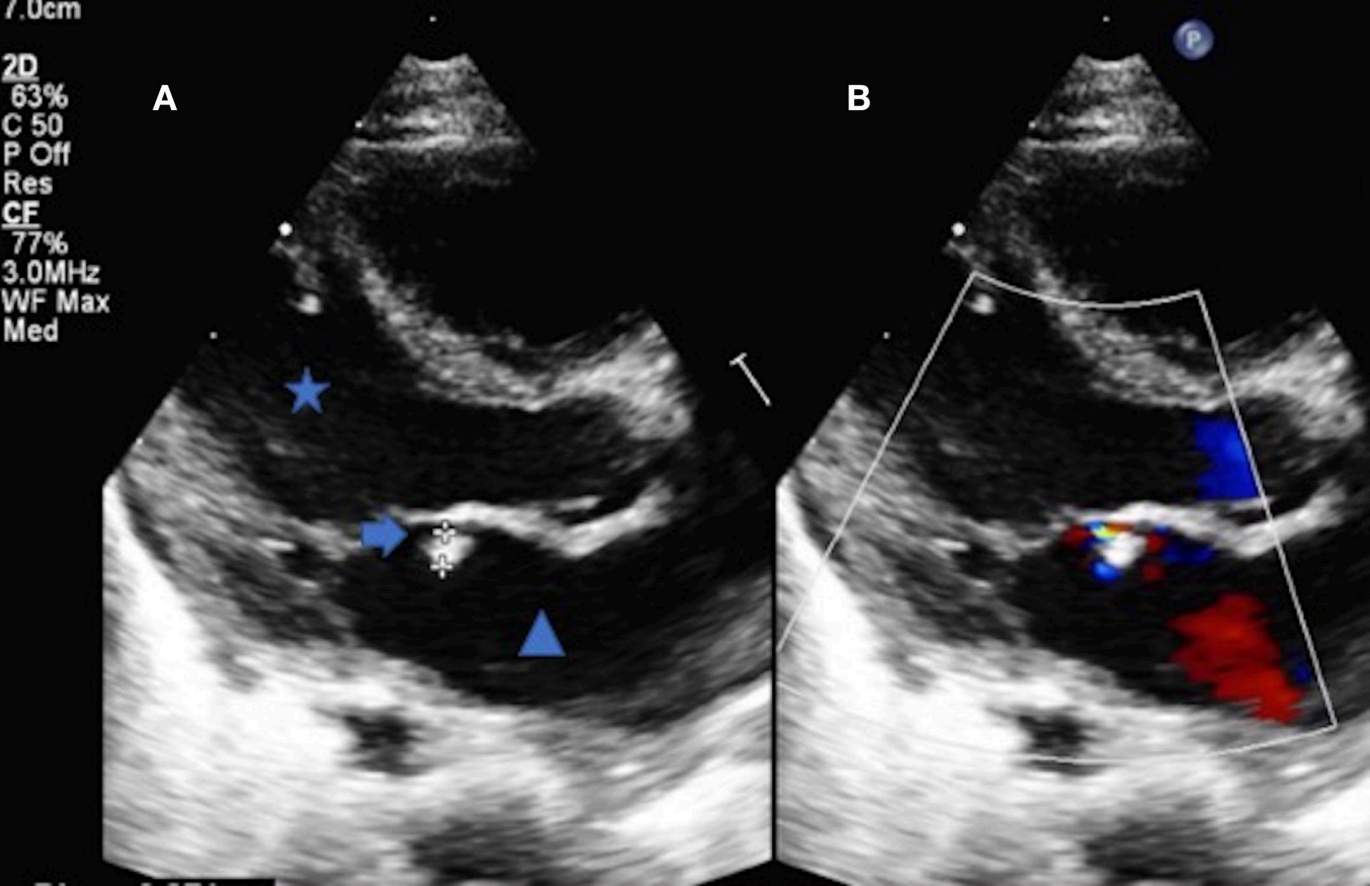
(A) Trans thoracic echocardiography parasternal long axis of the heart demonstrating the left ventricle (Star), left atrium (Triangle) and the mobile vegetation attached to the anterior leaflet of the mitral valve (Arrow). The vegetation measured 2.7 mm (B) The color Doppler comparison demonstrates the regurgitation from the anterior leaflet of the mitral valve secondary to the vegetation.
